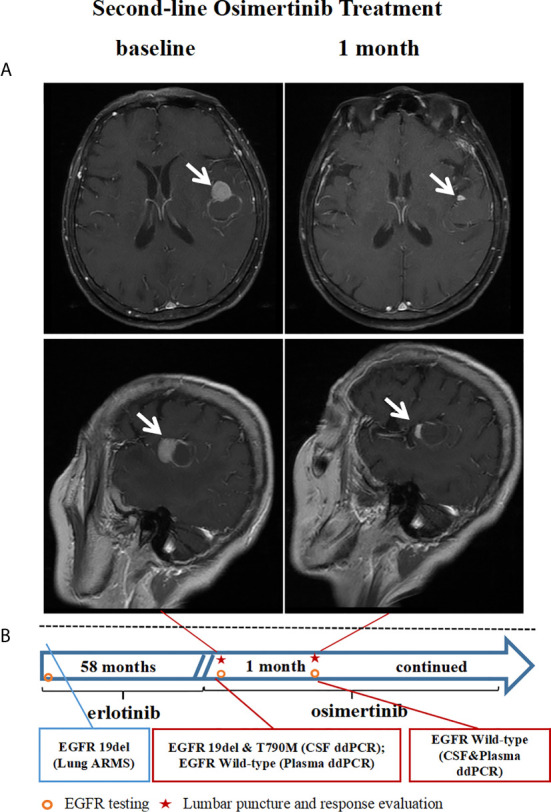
Case presentation: A case received second-line osimertinib treatment after a CSF EGFR T790M mutation was identified by ddPCR. (A) Brain MRI imaging tests before and after osimertinib treatment. (B) The timeline and results of EGFR mutation status identified by ddPCR. CSF, cerebrospinal fluid; EGFR, epidermal growth factor receptor; T790M, an amino acid substitution at position 790 in EGFR from a threonine to a methionine; ddPCR, droplet digital PCR; MRI, magnetic resonance imaging.
